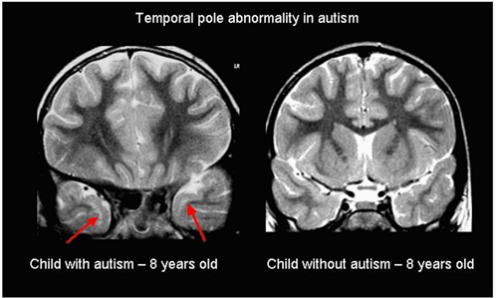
Temporal pole abnormalities in a child with autism. Example of a typical sub-cortical hyperintensities on T2-weighted coronal images localized in the temporal poles observed in children with autism (red arrows) and a normal image of a control child without autism.
